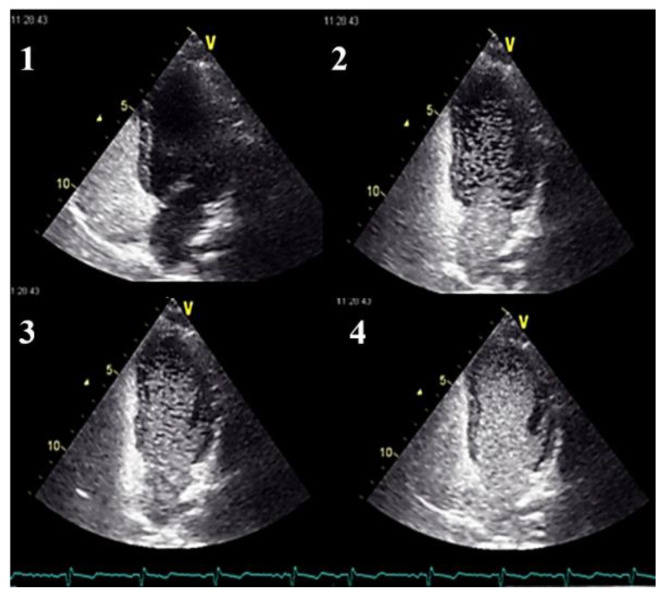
Contrast echocardiography showing progressive opacification with micro-bubbles of the left cavities in images 1 through 4, due to right-to-left intrapulmonary shunting. See also Supplementary Material—Video S1.
